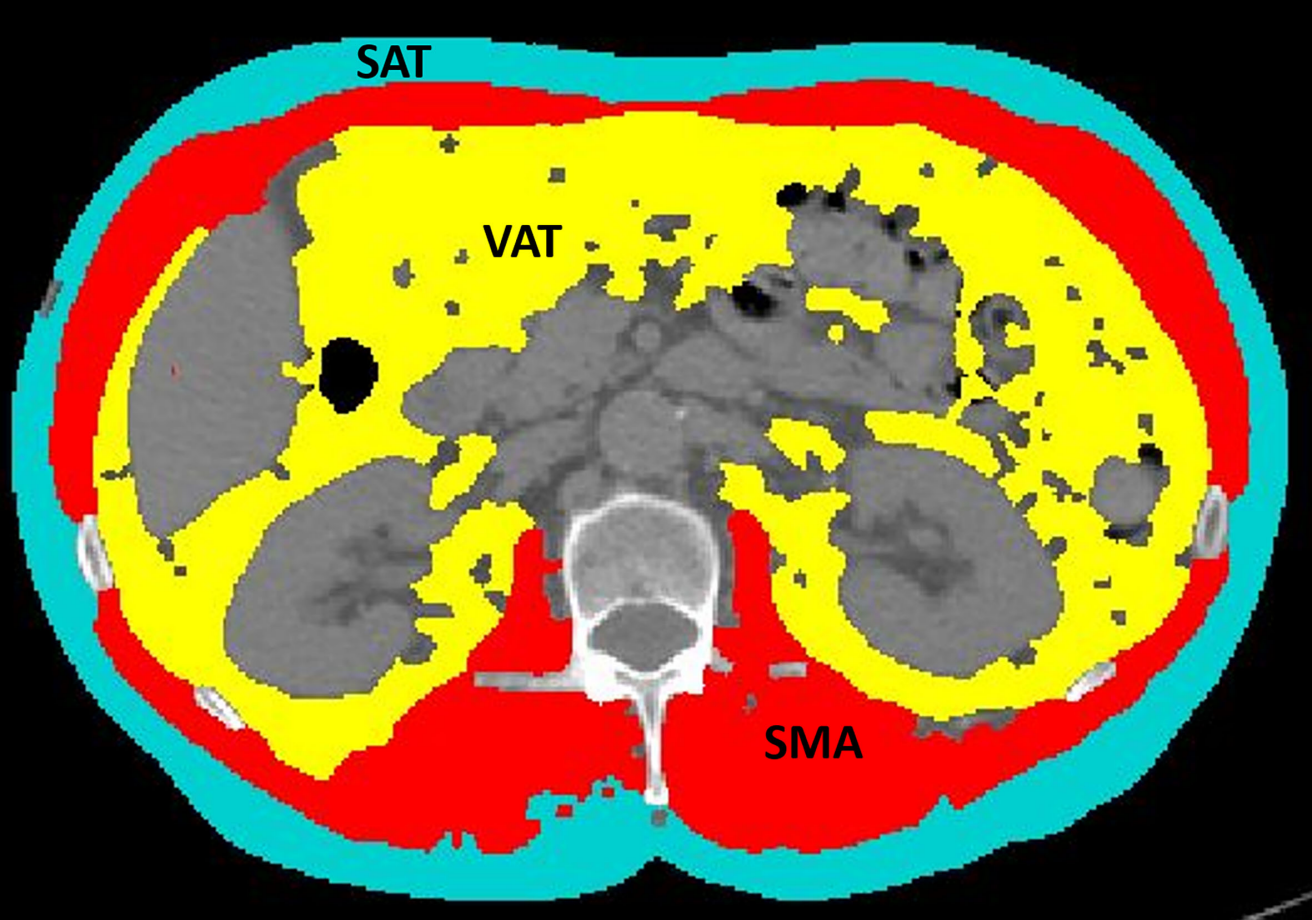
An example of segmentation of skeletal muscle area (red), visceral adipose tissue (yellow), and subcutaneous adipose tissue (light blue) from an axial image at the level of L3 of a CT scan performed as routine pretreatment staging. The colored parts are quantified as areas (cm^2^) by the software. The excluded parts include abdominal organs, such as liver, kidneys, and bowel (dark gray), as well as bones, such as vertebral body and ribs (light gray and white).
